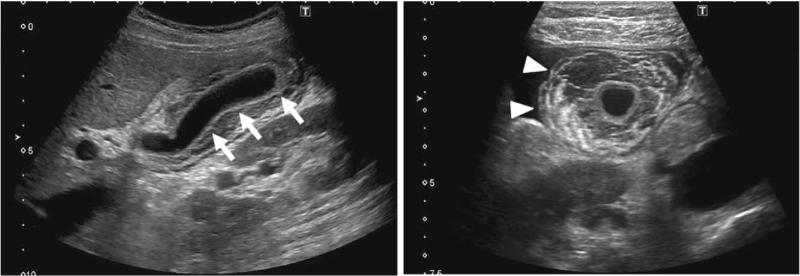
Abdominal ultrasonography. Edematous wall thickening of the gallbladder is detected (12.0 mm) (white arrow), and the inner cavity is not enlarged, although the gallbladder is enlarged in diameter (44.0 mm). In addition, edematous wall thickening of the gallbladder was observed, accompanied by subserosal edema (white arrowhead).
